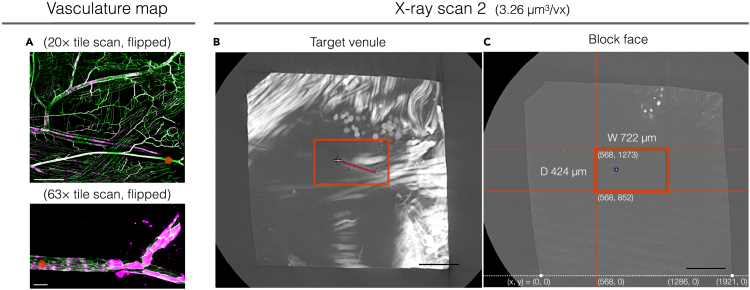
Annotations for Trimming 2 guided by vasculature maps and X-ray scan 2 (A) Vasculature maps with position of the ROI indicated by red dots. (B) A slice parallel to the block face plane in the X-ray scan 2 data showing the target venule. White cross indicates the position of the ROI identified in the X-ray scan 2 based on the distance of the ROI from the branch point of the Y-shaped venule. The area for the final block face is shown by a red rectangle. (C) Block face plane in the X-ray scan 2 data. The area for the final block face is shown by a red rectangle in the center. The positions for trimming 2 were determined by measuring distances from block corners as indicated. Scale bars: (A) 500 μm (top) and 50 μm (bottom); (B, C) 400 μm.c.Create 3dmod snapshots of slices at the block face and at the plane containing the ROI together with the annotation (Figures 7B and 7C).d.In the snapshot of the block face plane, measure the position of the final block face using block corners.e.At an ultramicrotome, mount the sample and place a 90° trimming diamond knife at a knife angle 0°.f.Orient the block at an angle required for the trimming and align the block face to the cutting plane.g.At the leading edge of the block face, bring the knife edge of the 90° trimming diamond knife to the position measured in Step 40d using the measuring mode of the Leica UC7 ultramicrotome.h.Trim the final block.*Note:* It is recommended to use a 90° trimming diamond knife to keep the size of the block face uniform during the SBF-SEM.
